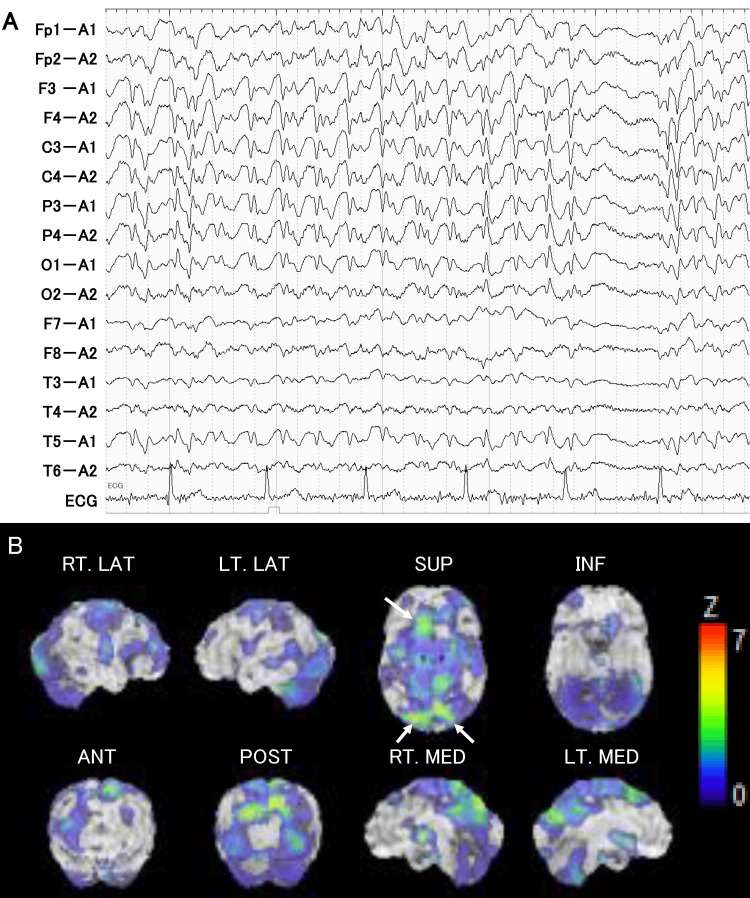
Neuroimaging of Case 2 at the second attack A: Electroencephalography six hours after onset, revealing spike and wave complex in the bilateral fronto-parieto-occipital lobes. B: Single-photon emission computed tomography on Day 1 revealing a high-uptake lesion in the bilateral superior parietal lobules (arrows) and left superior and middle frontal gyri (arrow).
